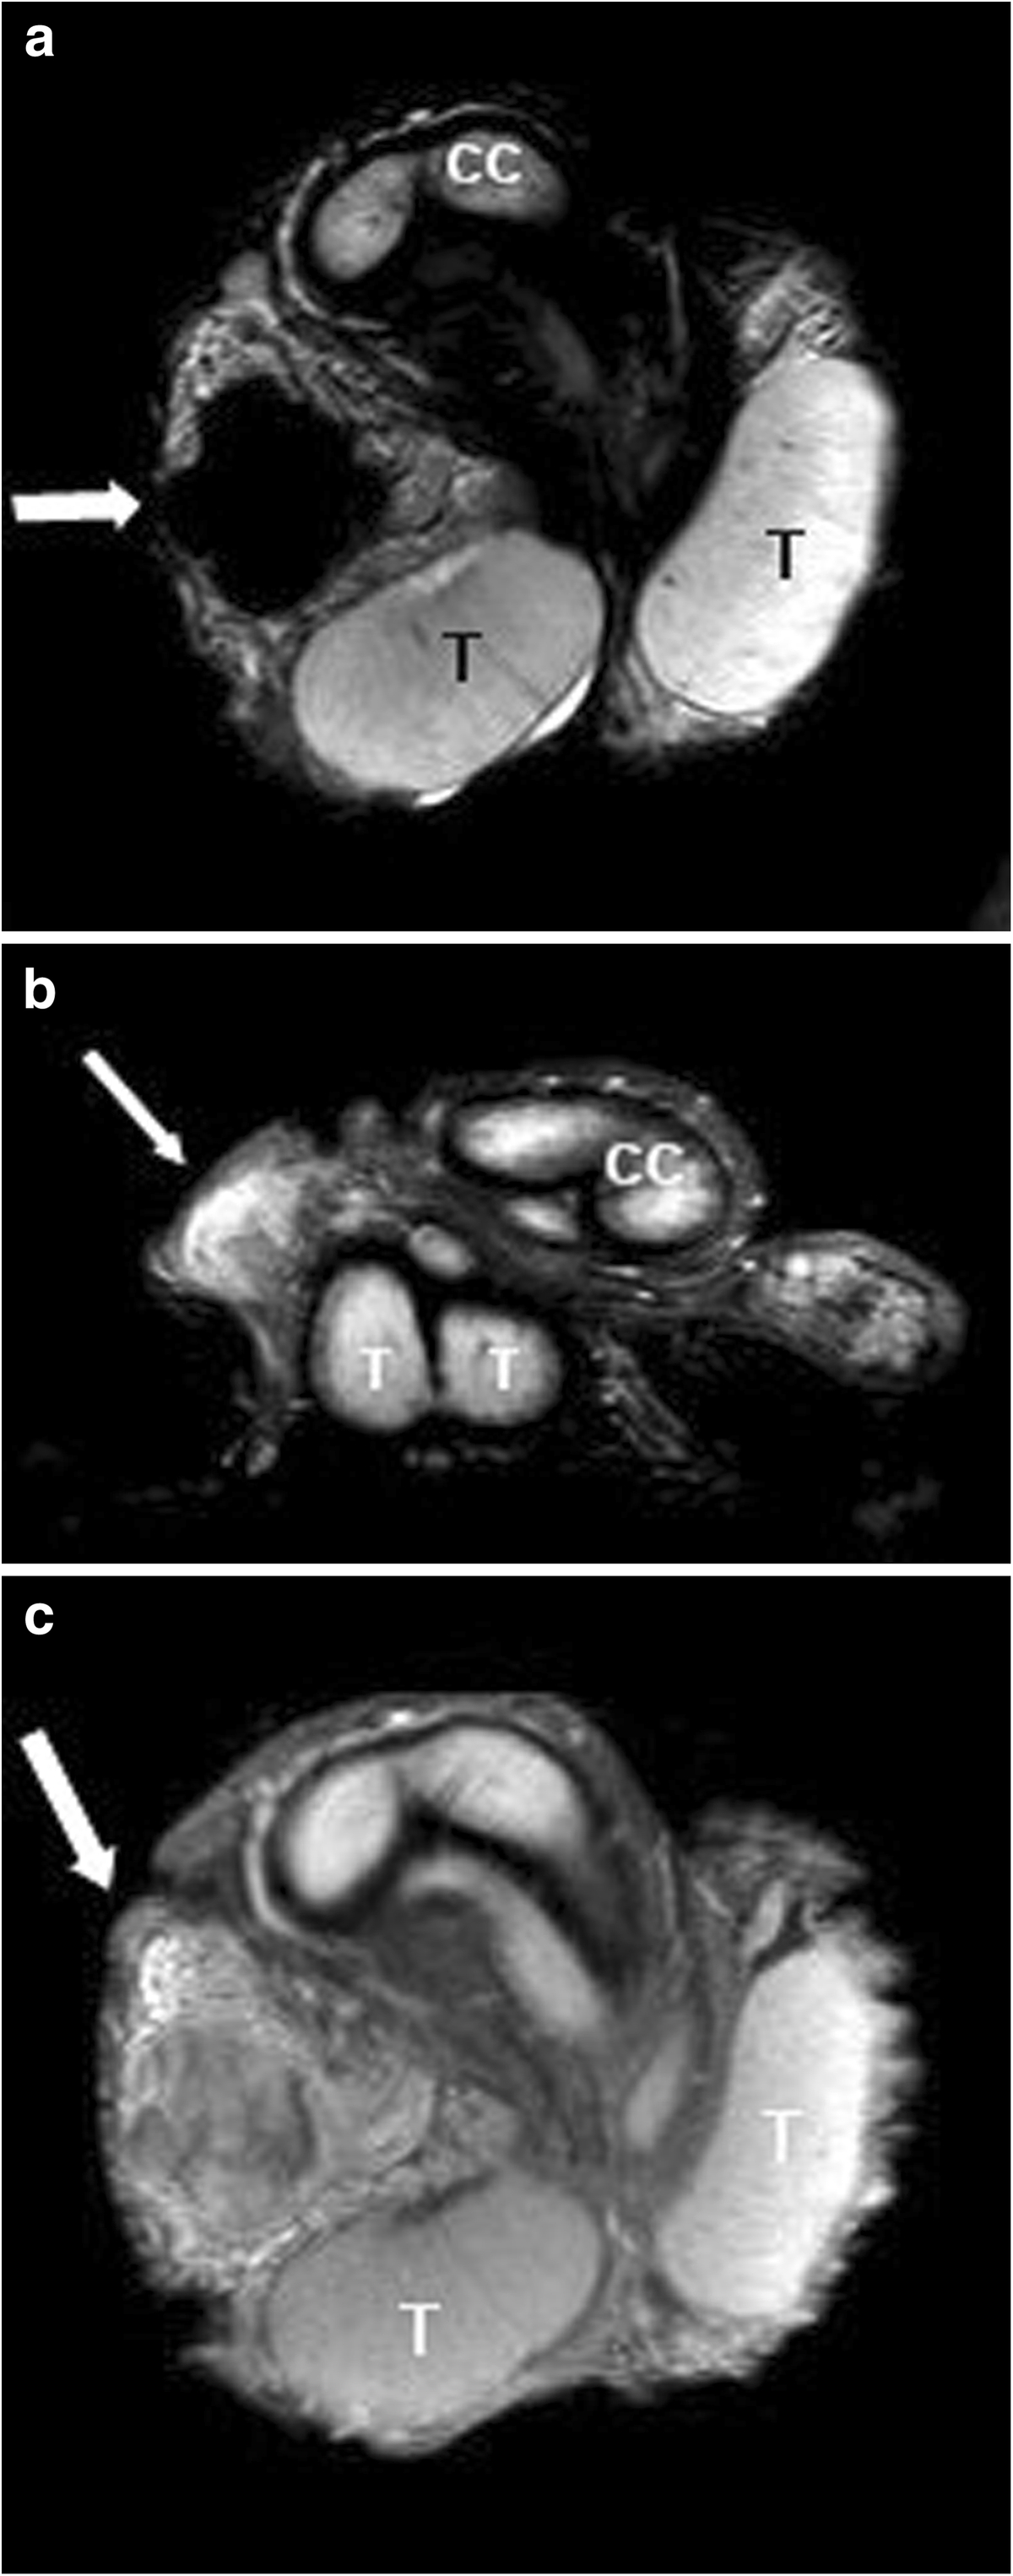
Magnetic resonance imaging (1.5 Tesla) with a surface coil. (a) Polycyclic mass (arrow) cephalad to the right testicle with no signal intensity on T2-weighted imaging. The mass is clearly detached from the testicle (coronary section). (b) The intrascrotal mass (arrow) shows inhomogeneous signal intensity on T1-weighted imaging (coronary section). (c) The mass (arrow) shows only low and inhomogeneous uptake of gadolinium contrast agent (coronary section). CC, corpus cavernosum; T, testicle.
